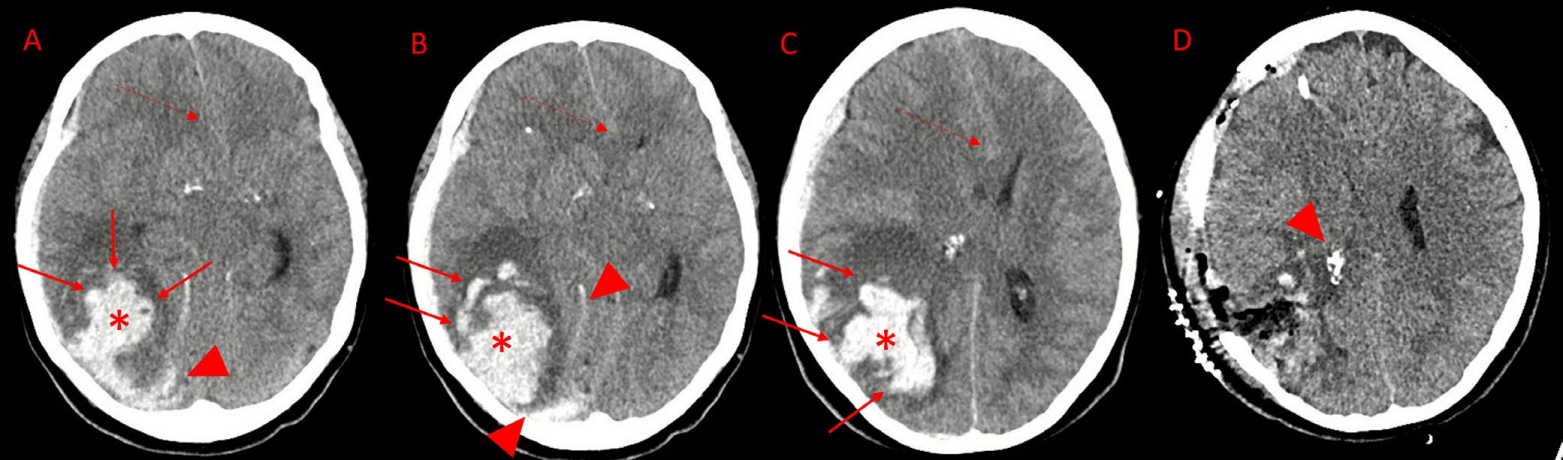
Preoperative and postoperative CT images. Unenhanced CT images show right occipital ICH (*) with fingerlike projections (arrows) and subarachnoid hemorrhage (SAH) (arrowheads) before surgery (A–C). Cerebral oedema and midline shift are evident. Post‐surgery CT (D) shows decompressive craniectomy, ICH evacuation, reduced oedema, and remnants of SAH (arrowhead).
